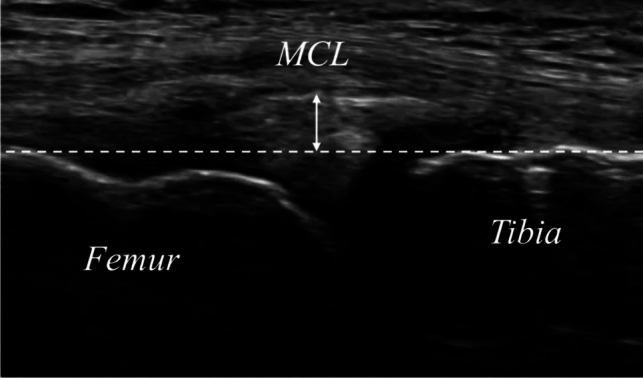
Ultrasonographic findings of medial meniscus extrusion Medial meniscal extrusion (MME) was evaluated on a longitudinal image that significantly depicted the medial collateral ligament (MCL). MME is a straight line parallel to the MCL passing through the outer margin of the tibia. MME was defined as the amount of perpendicular deviation from a straight line parallel to the MCL passing through the outer tibial border. In this study, MME was measured in both the supine and upright positions, with the difference defined as ΔMME (upright - supine)
